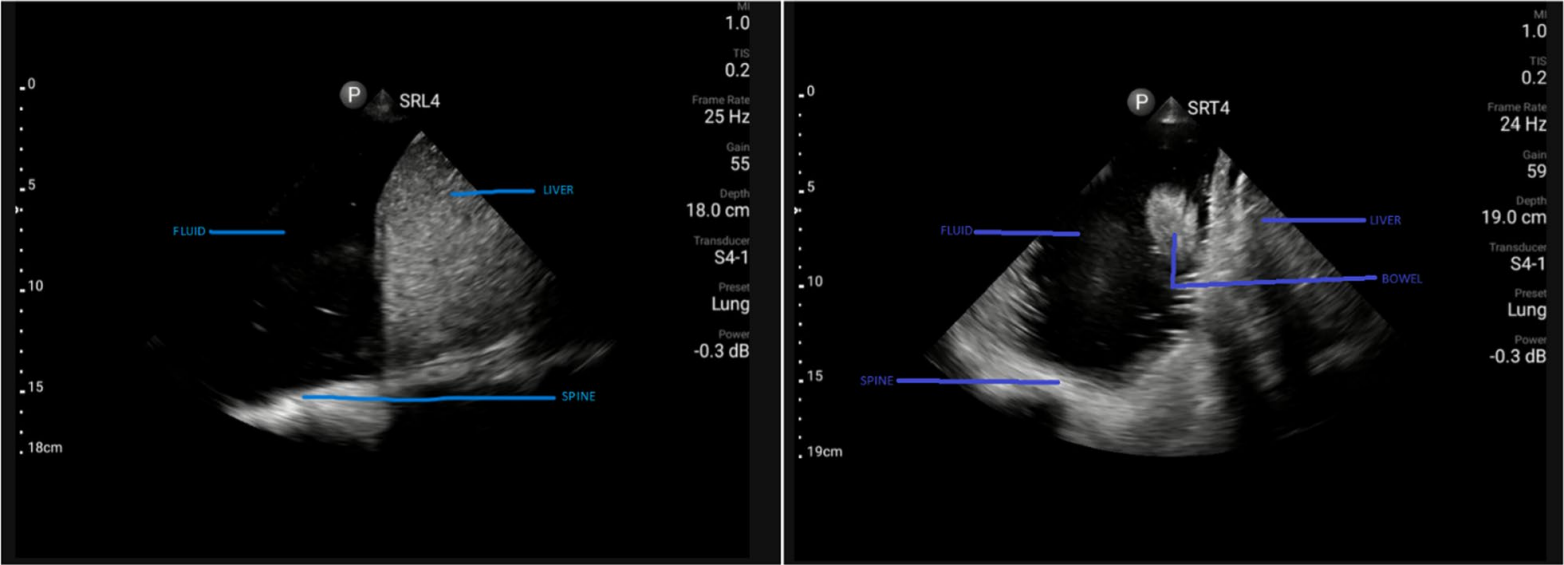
Point-of-care lung ultrasound right mid-axillary region on admission. A longitudinal view (left) and a transverse view (right) were taken
